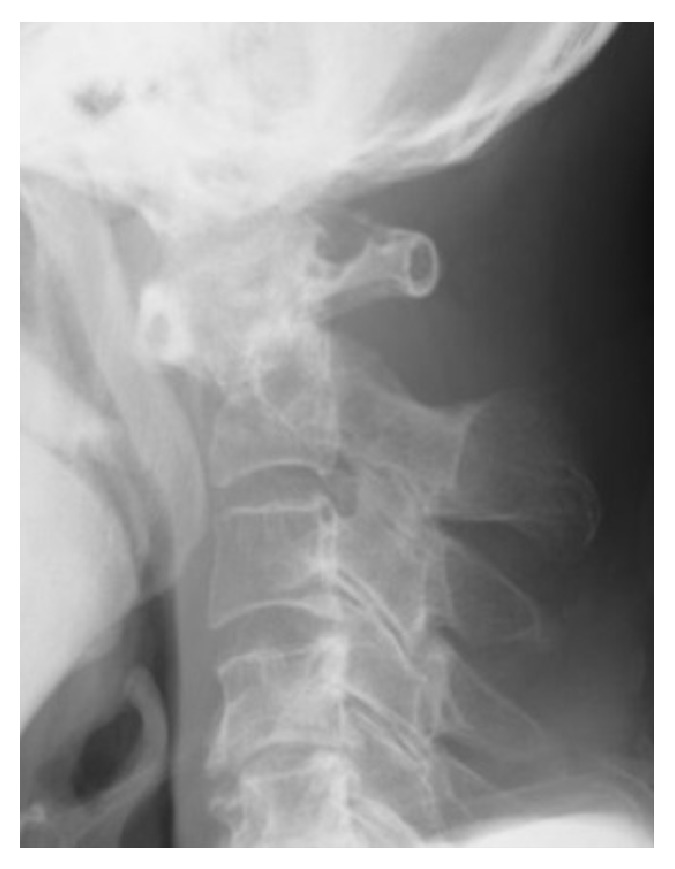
Lateral view of the cervical radiograph showing atlantoaxial subluxation in the flexion position.
